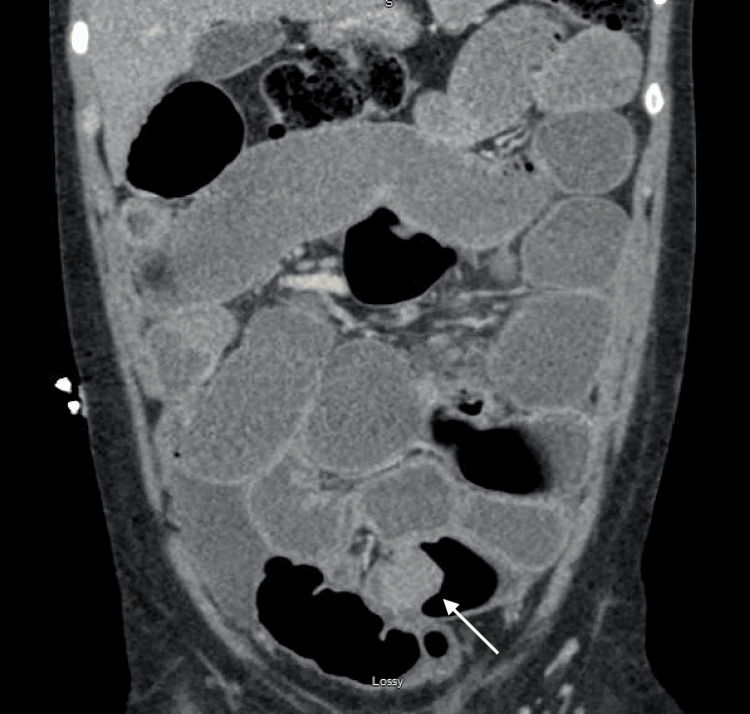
CT scan demonstrating diffusely dilated small bowel loops proximal to a transition point with associated enhancing nodularity (arrow) in the central anterior pelvis.
